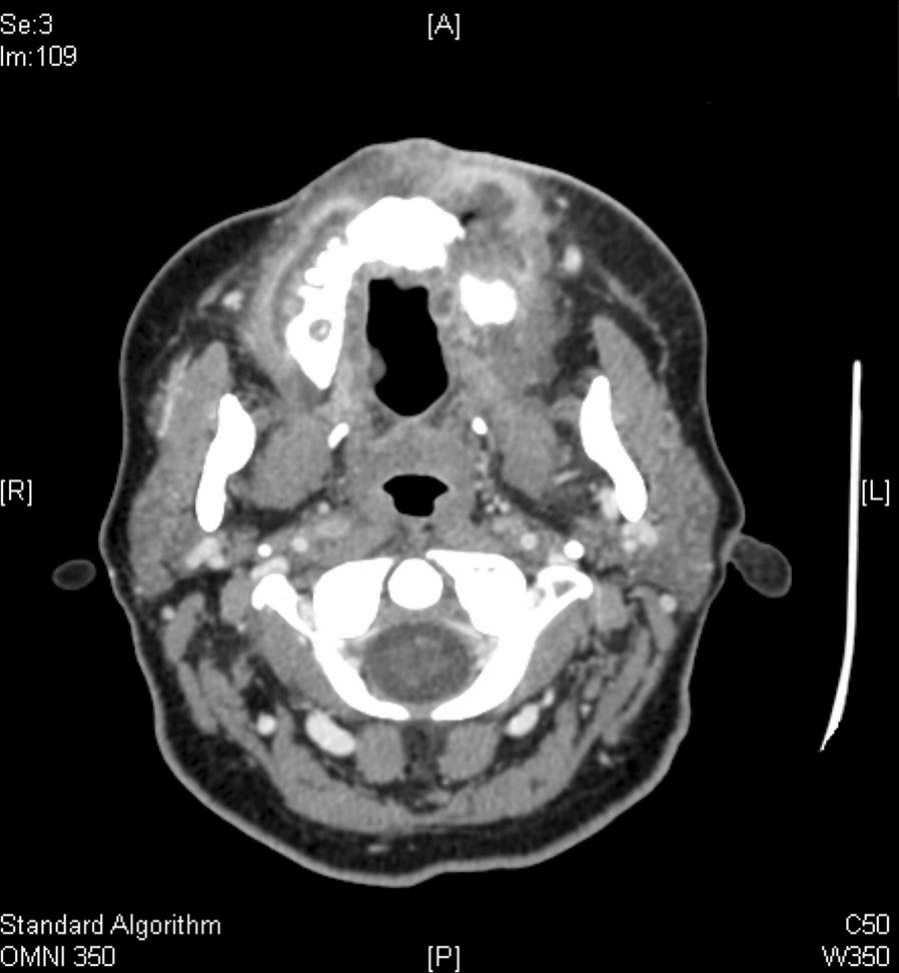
CT of neck demonstrating prominent maxillary gingival and palatal swelling and ulcerations with significant loss of maxillary teeth on the left side
